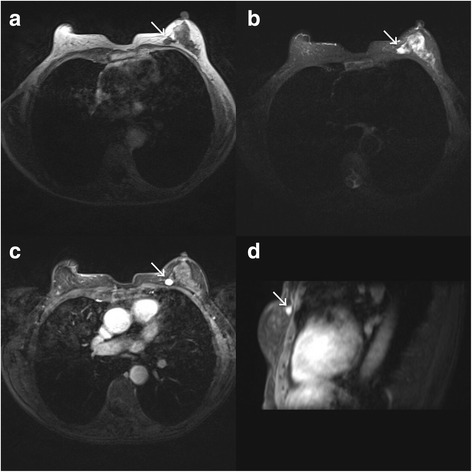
Breast MRI. Rounded lesion in the left breast was adjacent to the chest wall, hypo-intense on a T1-weighted image (a), hyperintense on a fat-saturated T2-weighted image (b) and was significantly enhanced following gadolinium administration (c and d)
